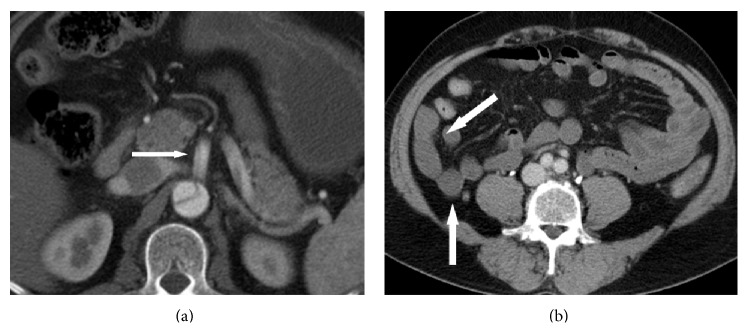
Contrast-enhanced CT shows (a) a type B aortic dissection, dissected SMA, and compressed true lumen (arrow) and (b) poor contrast enhancement of some of the small intestines (arrow).
